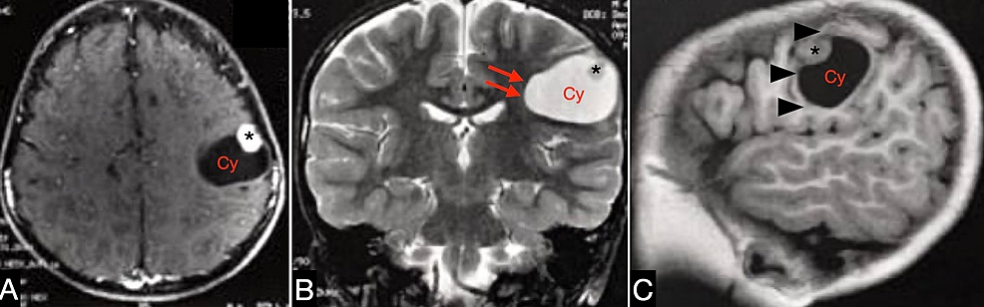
Imaging features of pleomorphic xanthoastrocytoma. The axial-weighted magnetic resonance imaging (MRI) scan after the addition of gadolinium shows a nonenhanced cystic lesion with an intensely enhanced mural nodule (*) in the left frontal lobe (A). The T2-weighted MRI scan in the coronal view shows the hyperintense cystic lesion (Cy) with an isointense mural nodule that led to a discrete mass effect in the surrounding frontal lobe (double red arrows) (B). The T1-weighted sagittal MRI scan shows the tumor occupying the postcentral gyri (triple arrowheads) on the left frontal lobe (C).
